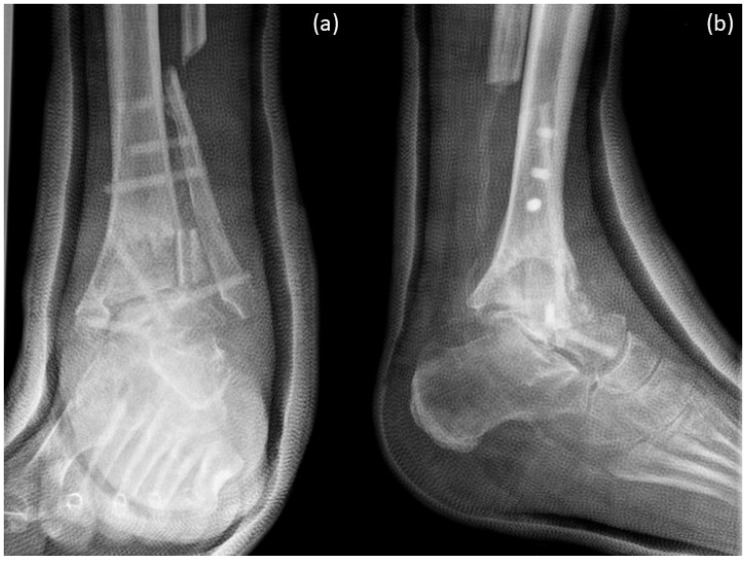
X-rays just after surgery. Arthrodesis was performed with 6 Shark Screws^®^ and a huge amount of allograft (femoral head and tricortical chip). (a) Ap-view; (b) lateral view.
